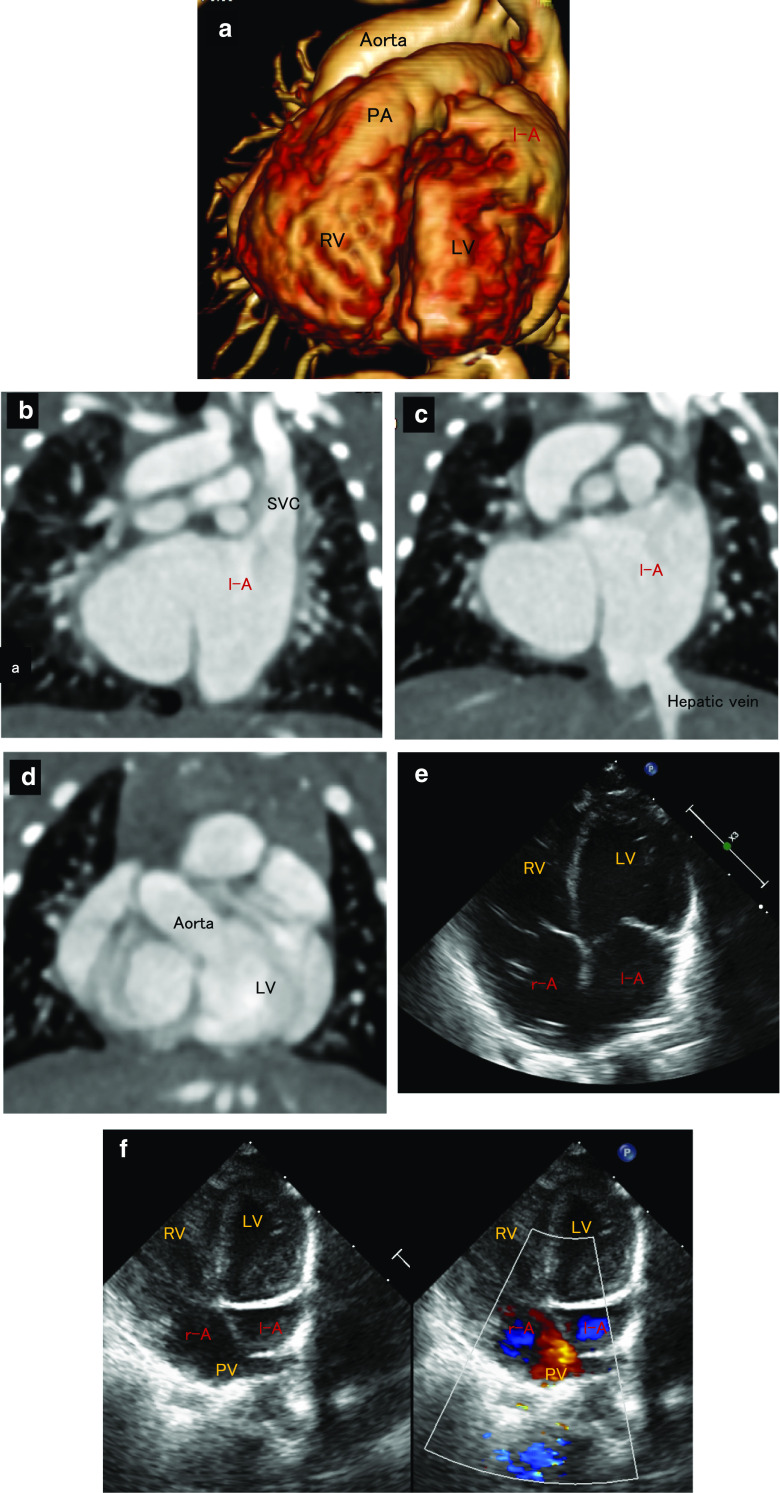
The computed tomography scans (a–d), and the transthoracic echocardiography (e, f) obtained from the patient. These figures indicate situs ambiguous(inversus), D-loop, and D-Spiral, atrioventricular discordance, ventriculoarterial concordance
